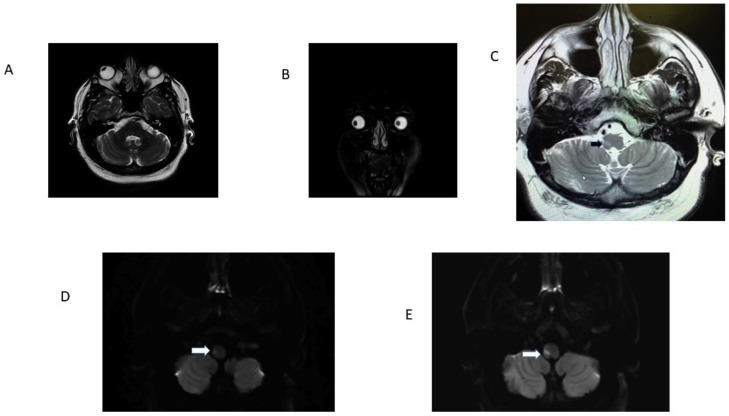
Patient 13 Table 1. Panel (A): note radiographic conjugate right ocular deviation, it correlated with clinical right OLD; panel (B) is a coronal T2-weighed MRI. Observe radiographic rightward deviation of the eyes in the coronal plane. Panel (C) is a T2 FLAIR image that identifies the precise location of the ischemic stroke (black arrow). Panels (D) and (E) are adjacent axial DWI scans. Note infarcts involving the right LVST and ICP responsible for OLD (white arrows). The ADC map confirmed diffusion restriction.
